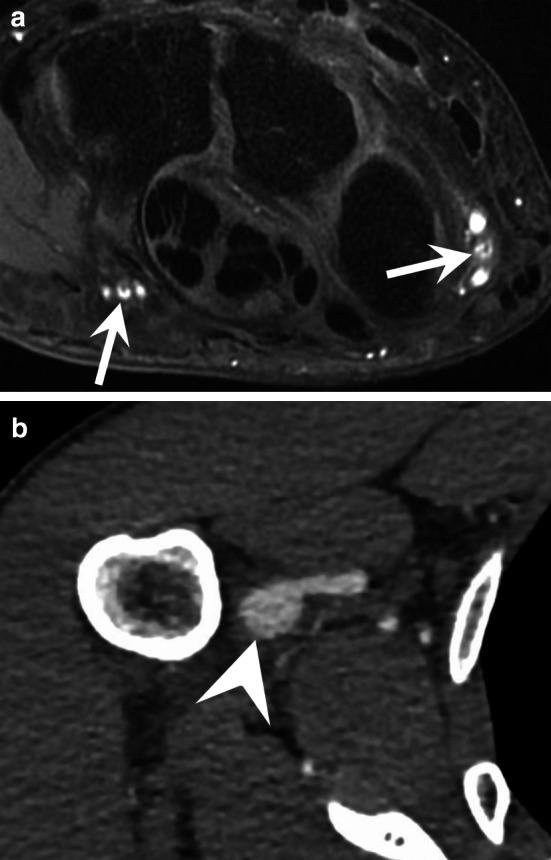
Distal emboli due to an aneurysm of the axillary artery in a 29-year-old female professional volleyball player. a Axial contrast-enhanced T1-weighted fat-suppressed sequence showing some emboli in the UA and RA (arrows). b CTA of the entire upper limb showing an aneurysm of the axillary artery
